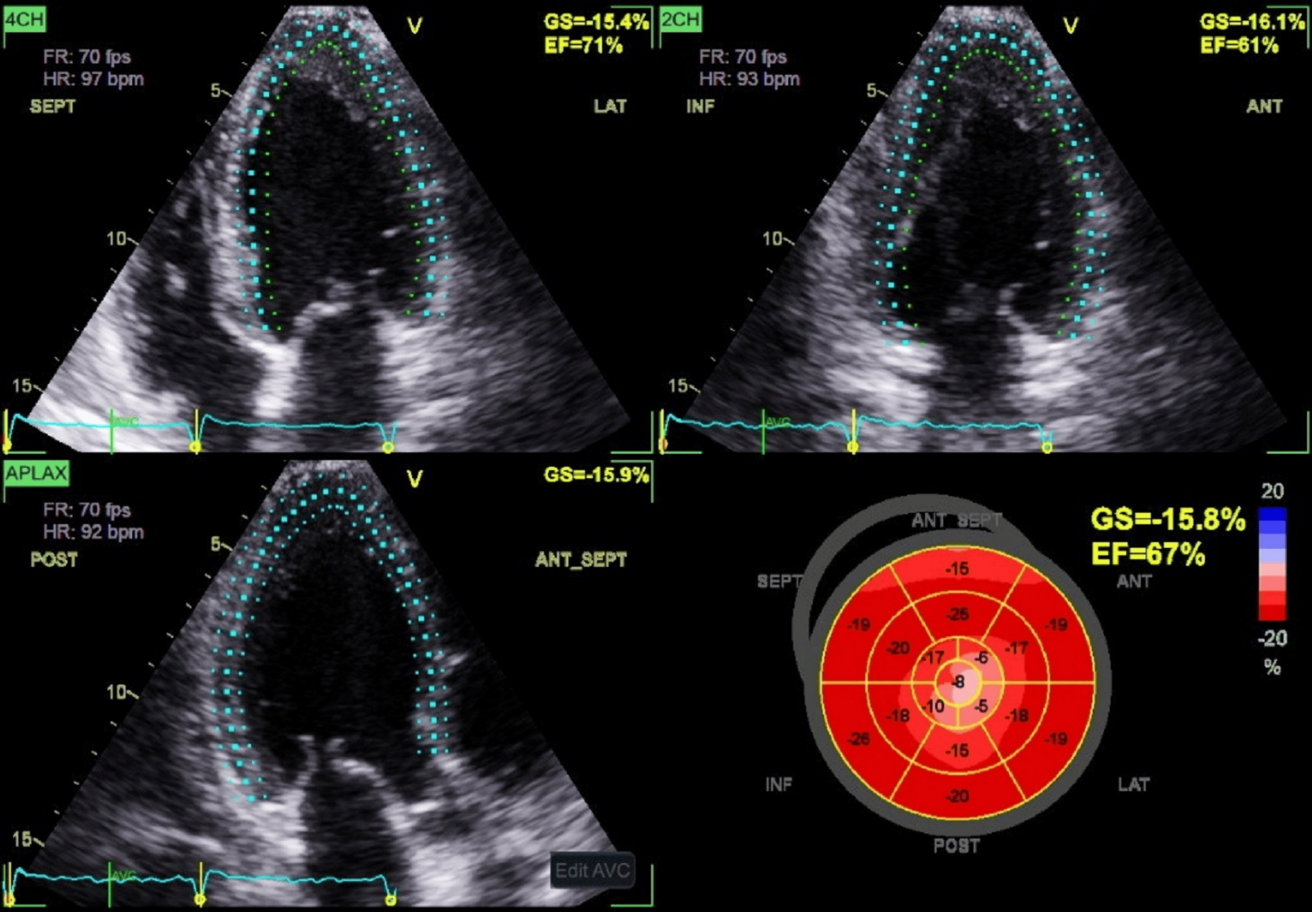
TTE showing marked reduction of the GLS at the apex TEE: trans-thoracic echocardiography, GLS: global longitudinal strain
